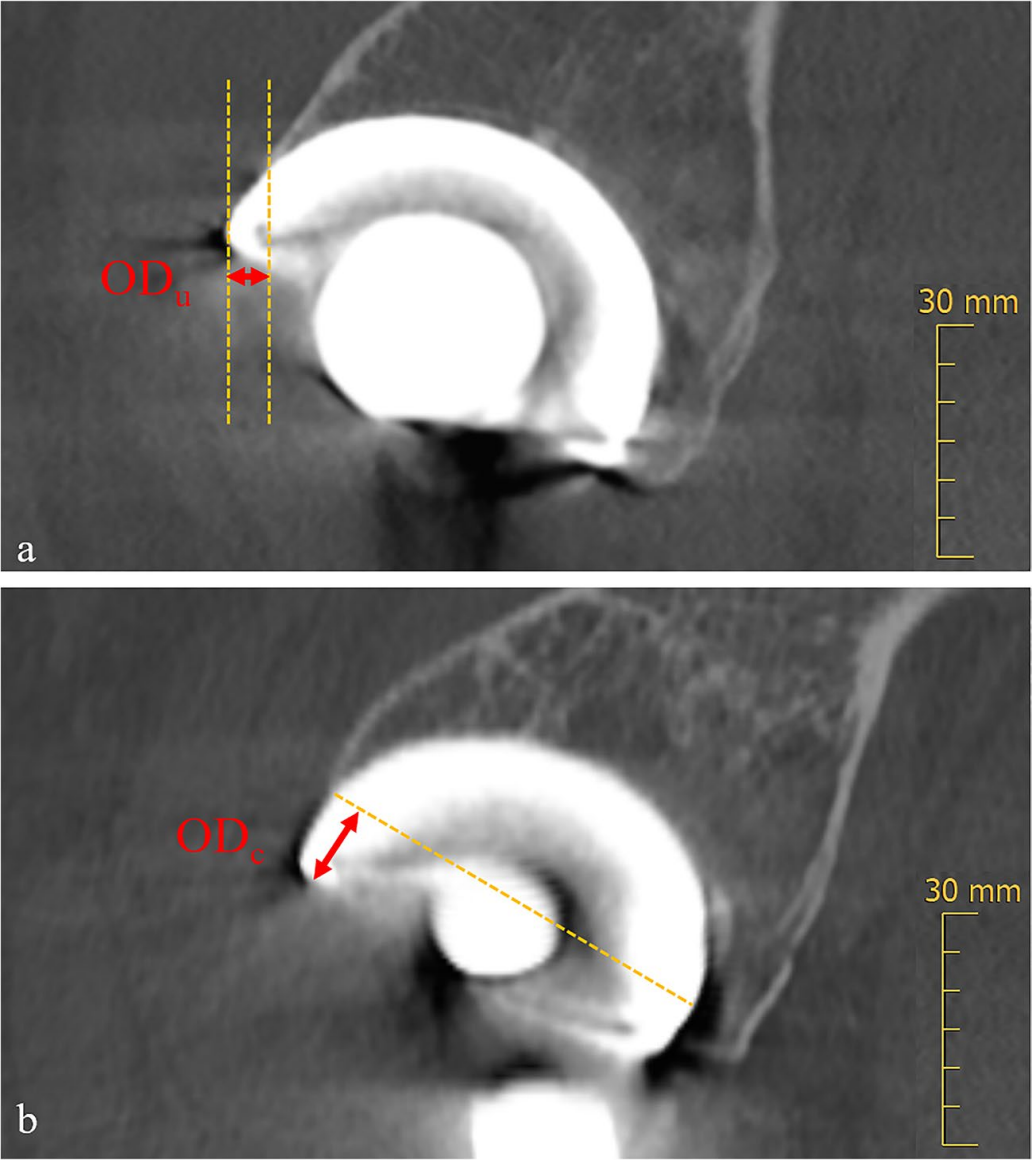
The orthogonal sagittal CT image of a patient with cup overhang without multiplanar reformation (MPR): This uncorrected overhang distance (double arrows, OD_u_) is measured according to the current literature (a). After MPR-aided correction for pelvic rotation and with coronal MPR angled at the plane of the cup face (b), the measurement of the overhang distance perpendicular to the cup circumference is shown in the same patient (double arrows, OD_c_). All measurements were carried out at the slice in which the maximum distance of uncovered cup was visible
